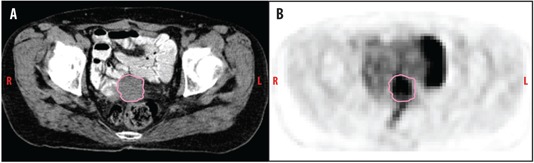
Postchemotherapy (a) axial CT and (b) PET image of the pelvis shows a residual 3.3 cm prerectal/primary peritoneal clear cell carcinoma with FDG activity in the posterior vagina.
